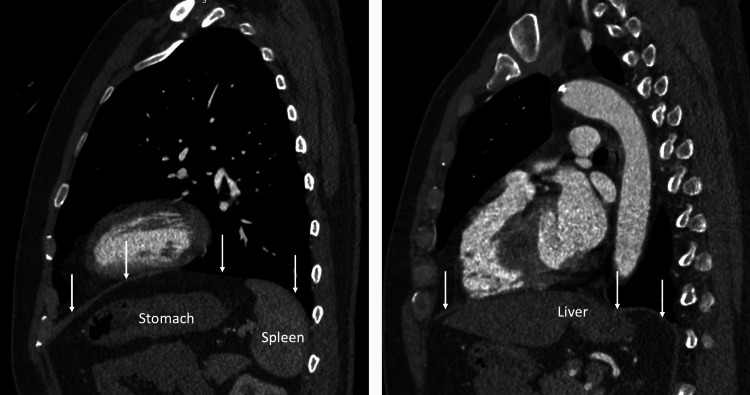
CT examples of the diaphragmatic region in the sagittal plane noting the absence of pathology in this region. Arrows: diaphragm
